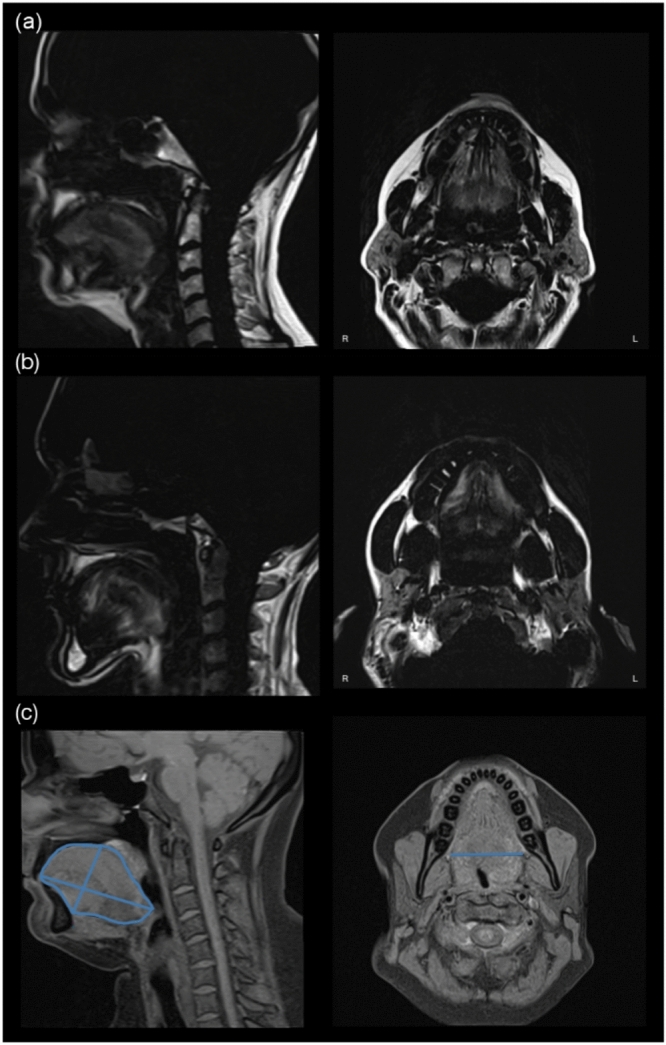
Magnetic resonance imaging of the tongue. MRI of the tongue in sagittal and transverse planes in a patient with SMA type 3 (a) and a patient with SMA type 2 (b) in a Dixon in-phase sequence. The tongue showing axial, anterior–posterior, midsagittal diameters, and midsagittal area (c) in a T1-weighted sequence
